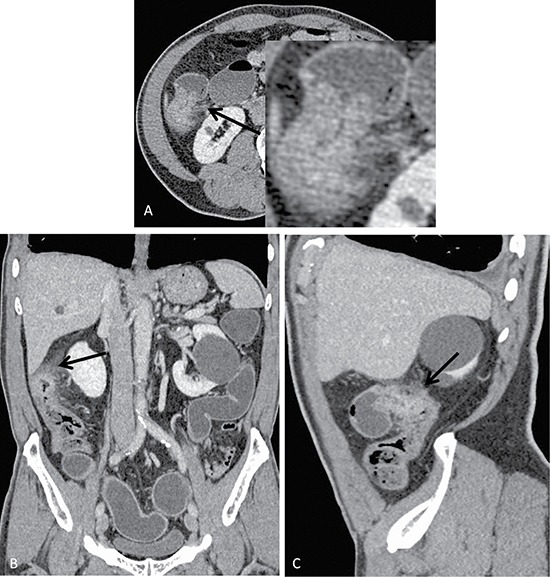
Axial (A), coronal (B) and sagittal (C) images of a colon cancer lesion located in the hepatic flexure of the colon of a 72-year-old male who presented with a change in defecation habits. Conventional methods preoperatively predicted the T-stage as T4 because of evident serosa thickening, increased surrounding fat gap density, a blurred neighboring peritoneal border, and adjacent peritoneal thickening on the CT image. Conversely, the model predicted T3 disease, which was consistent with the pathological staging.
